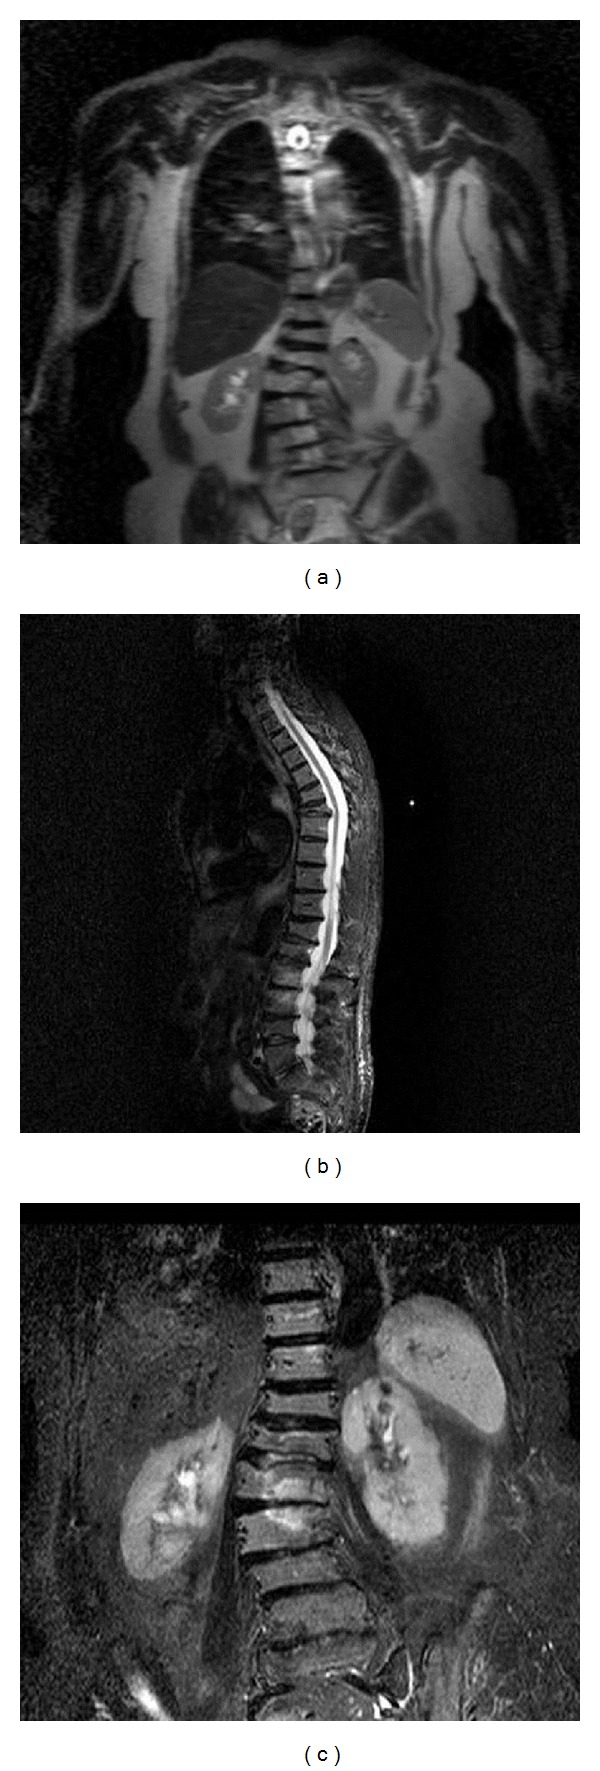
T2-weighted coronal sequence (a), sagital (b), and coronal (c) STIR sequence demonstrating scoliosis with the convex towards the right side and bone edema at L3, L3, and L1 vertebral bodies (in the inner side of the scoliotic arch). Notice the fracture line at L1 vertebral body under the upper end plate.
